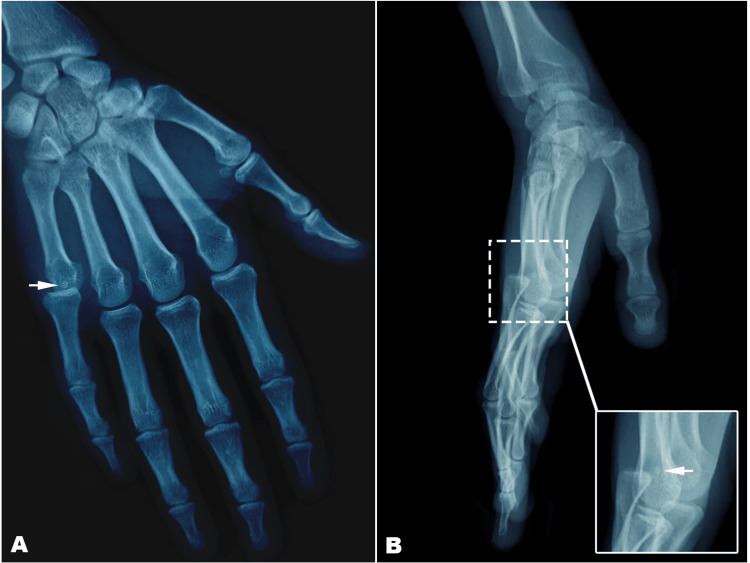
Preoperative X-rays of the right hand. (A) Anteroposterior view of the hand showed dorsal complex metacarpophalangeal joint dislocation of the small finger; (B) Lateral view of the hand showing the pure dislocation with no osteochondral fragment; sesamoid bone entrapment within joint (small white arrow).
